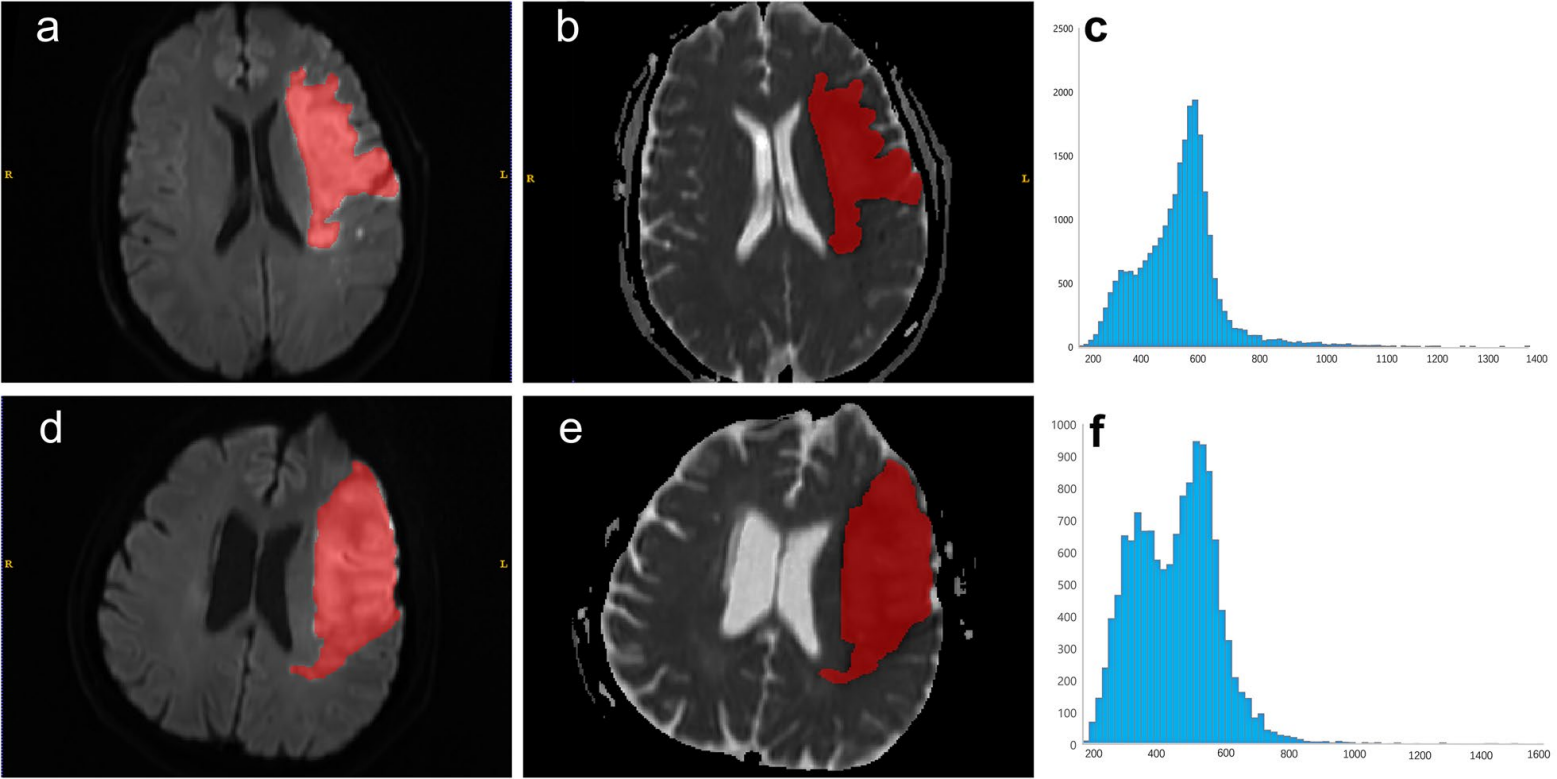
The patient was a 61-year-old male with subacute cerebral infarction in the left basal ganglia and the frontal lobe; the favorable outcome for day 90 mRS score is 2 (a–c). a Axial DWI (b = 1000) shows an obvious uniform hyperintense area (red), the infarct volume was 58.81 ml. b The ADC map shows an hypointense area within the diffusion-restricted region (red). c ADC histogram, the abscissa is the ADC value, and the ordinate is the pixel value. The patient was a 61-year-old male with a subacute cerebral infarction in the left basal ganglia and the frontal lobe; the unfavorable outcome for the 90-day mRS score is 3 (d–f). d Axial DWI (b = 1000) shows an obvious uniform hyperintense area (red), the infarct volume was 110.71 ml. e ADC map shows an hypointense area in the diffusion-restricted region (red). f. ADC histogram, the abscissa is the ADC value, and the ordinate is the pixel value
